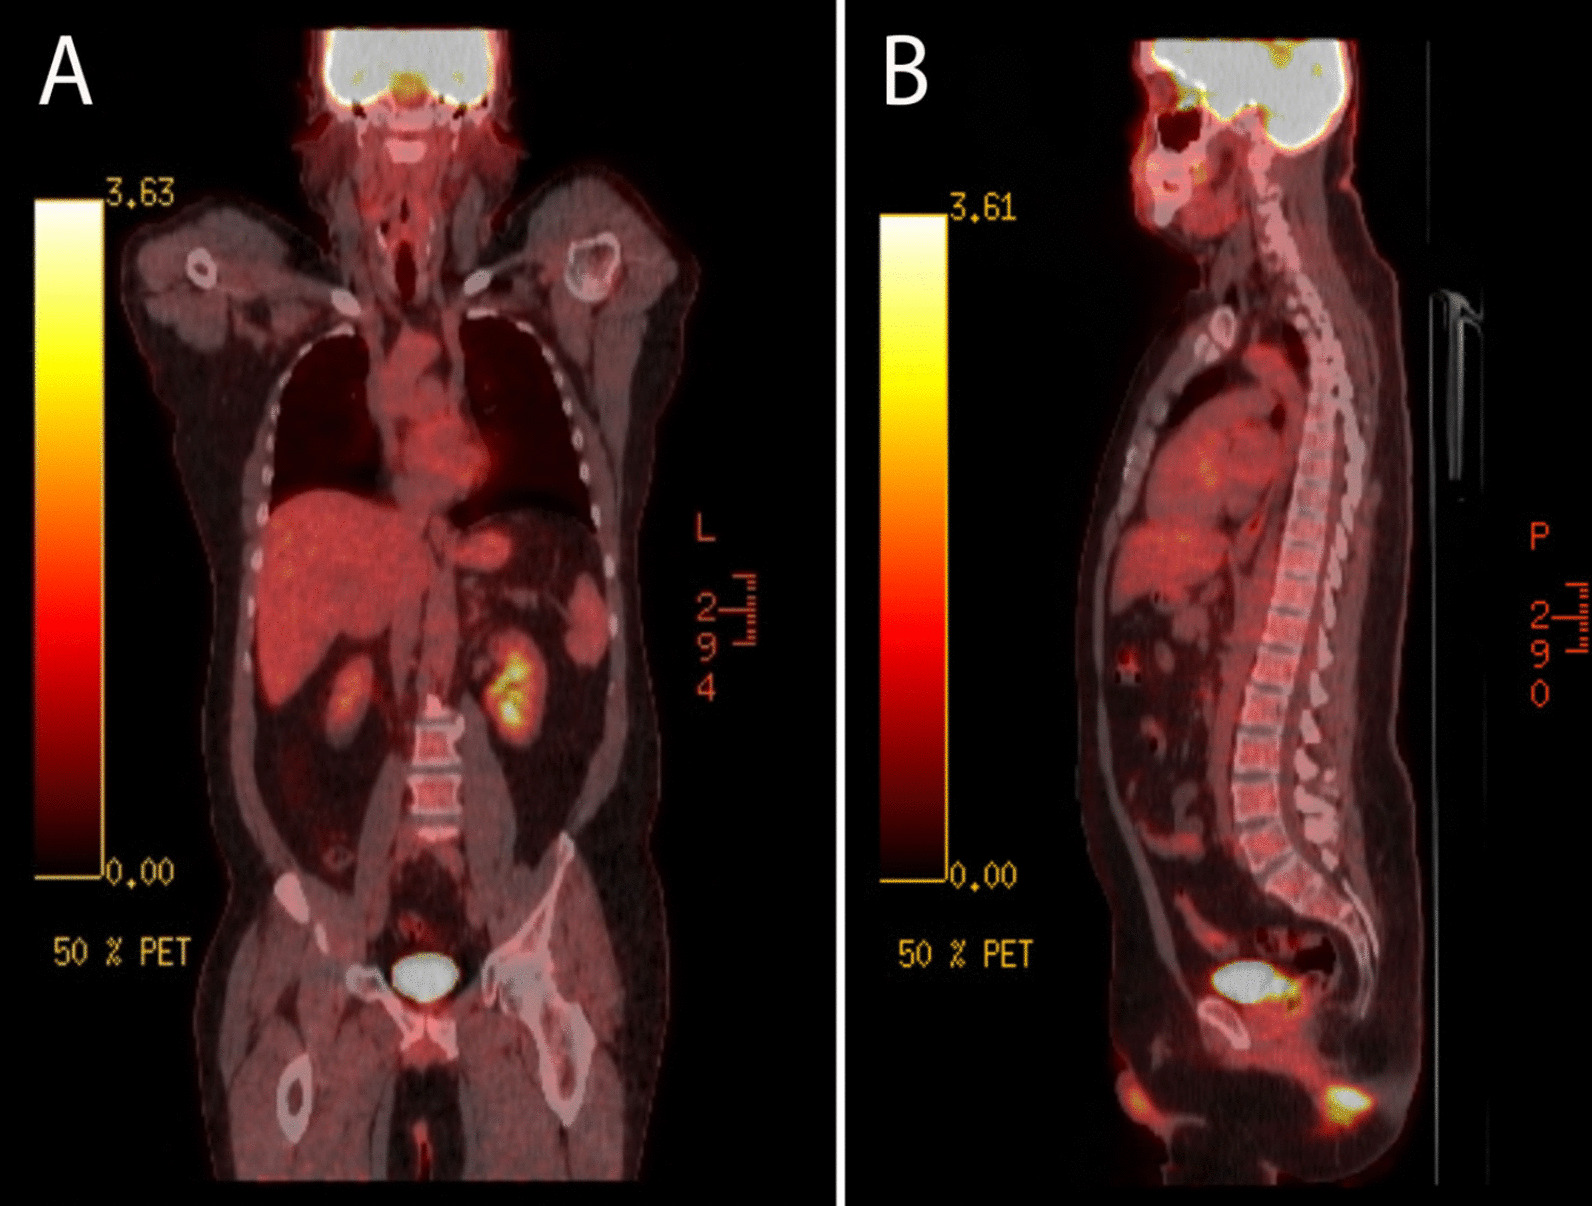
F-18 fluorodeoxyglucose (FDG)/PET scan in coronal (A) and sagittal views (B) showing physiological uptake
